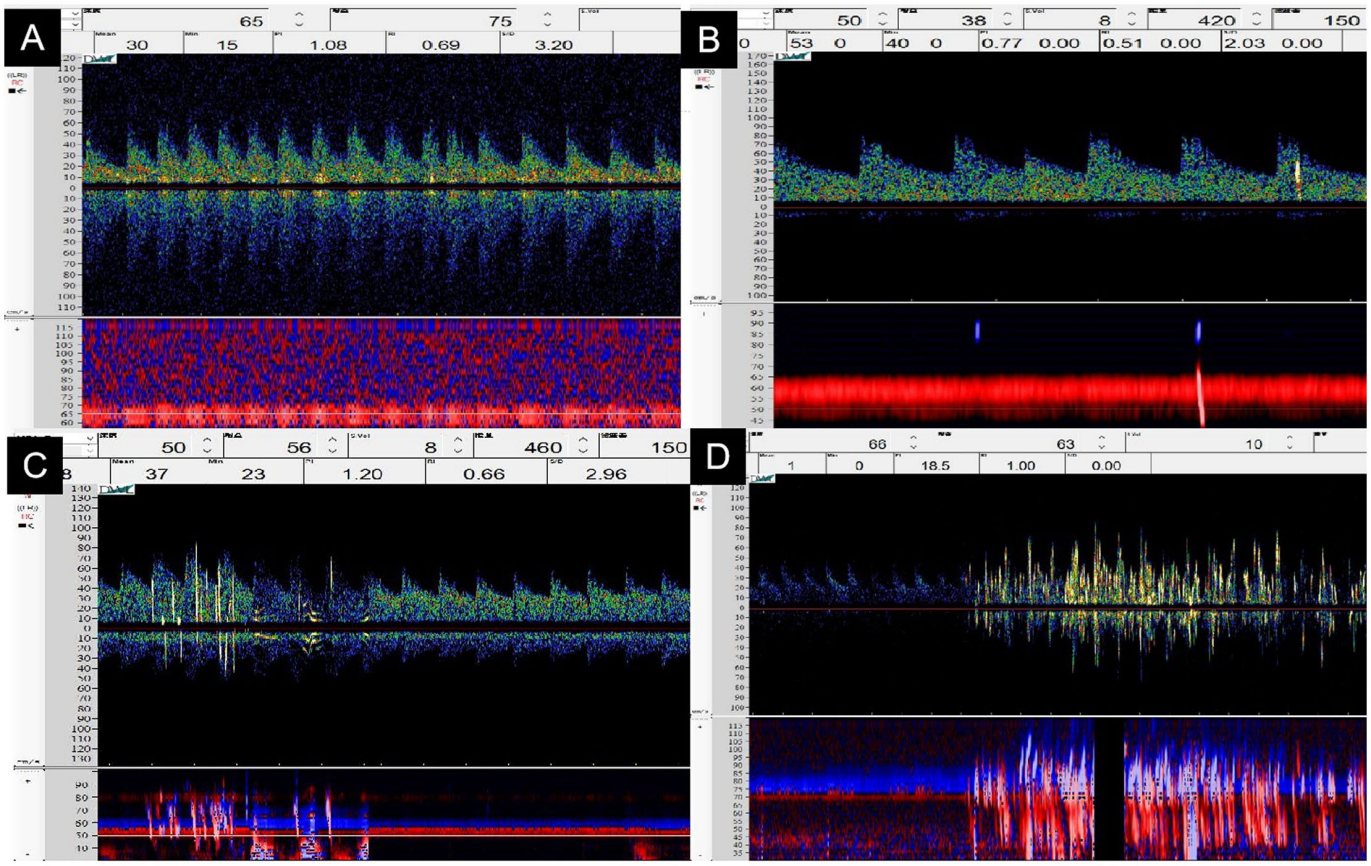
The grading classification of right-to-left shunts in contrast transcranial Doppler ultrasonography. (A) Grade 0_TCD_ = no microbubbles. (B) Grade I_TCD_ = 1–10 microbubbles (arrow). (C) Grade II_TCD_ = 10–30 microbubbles but no curtain (arrow). (D) Grade III_TCD_ = curtain-like microbubbles (arrow).
